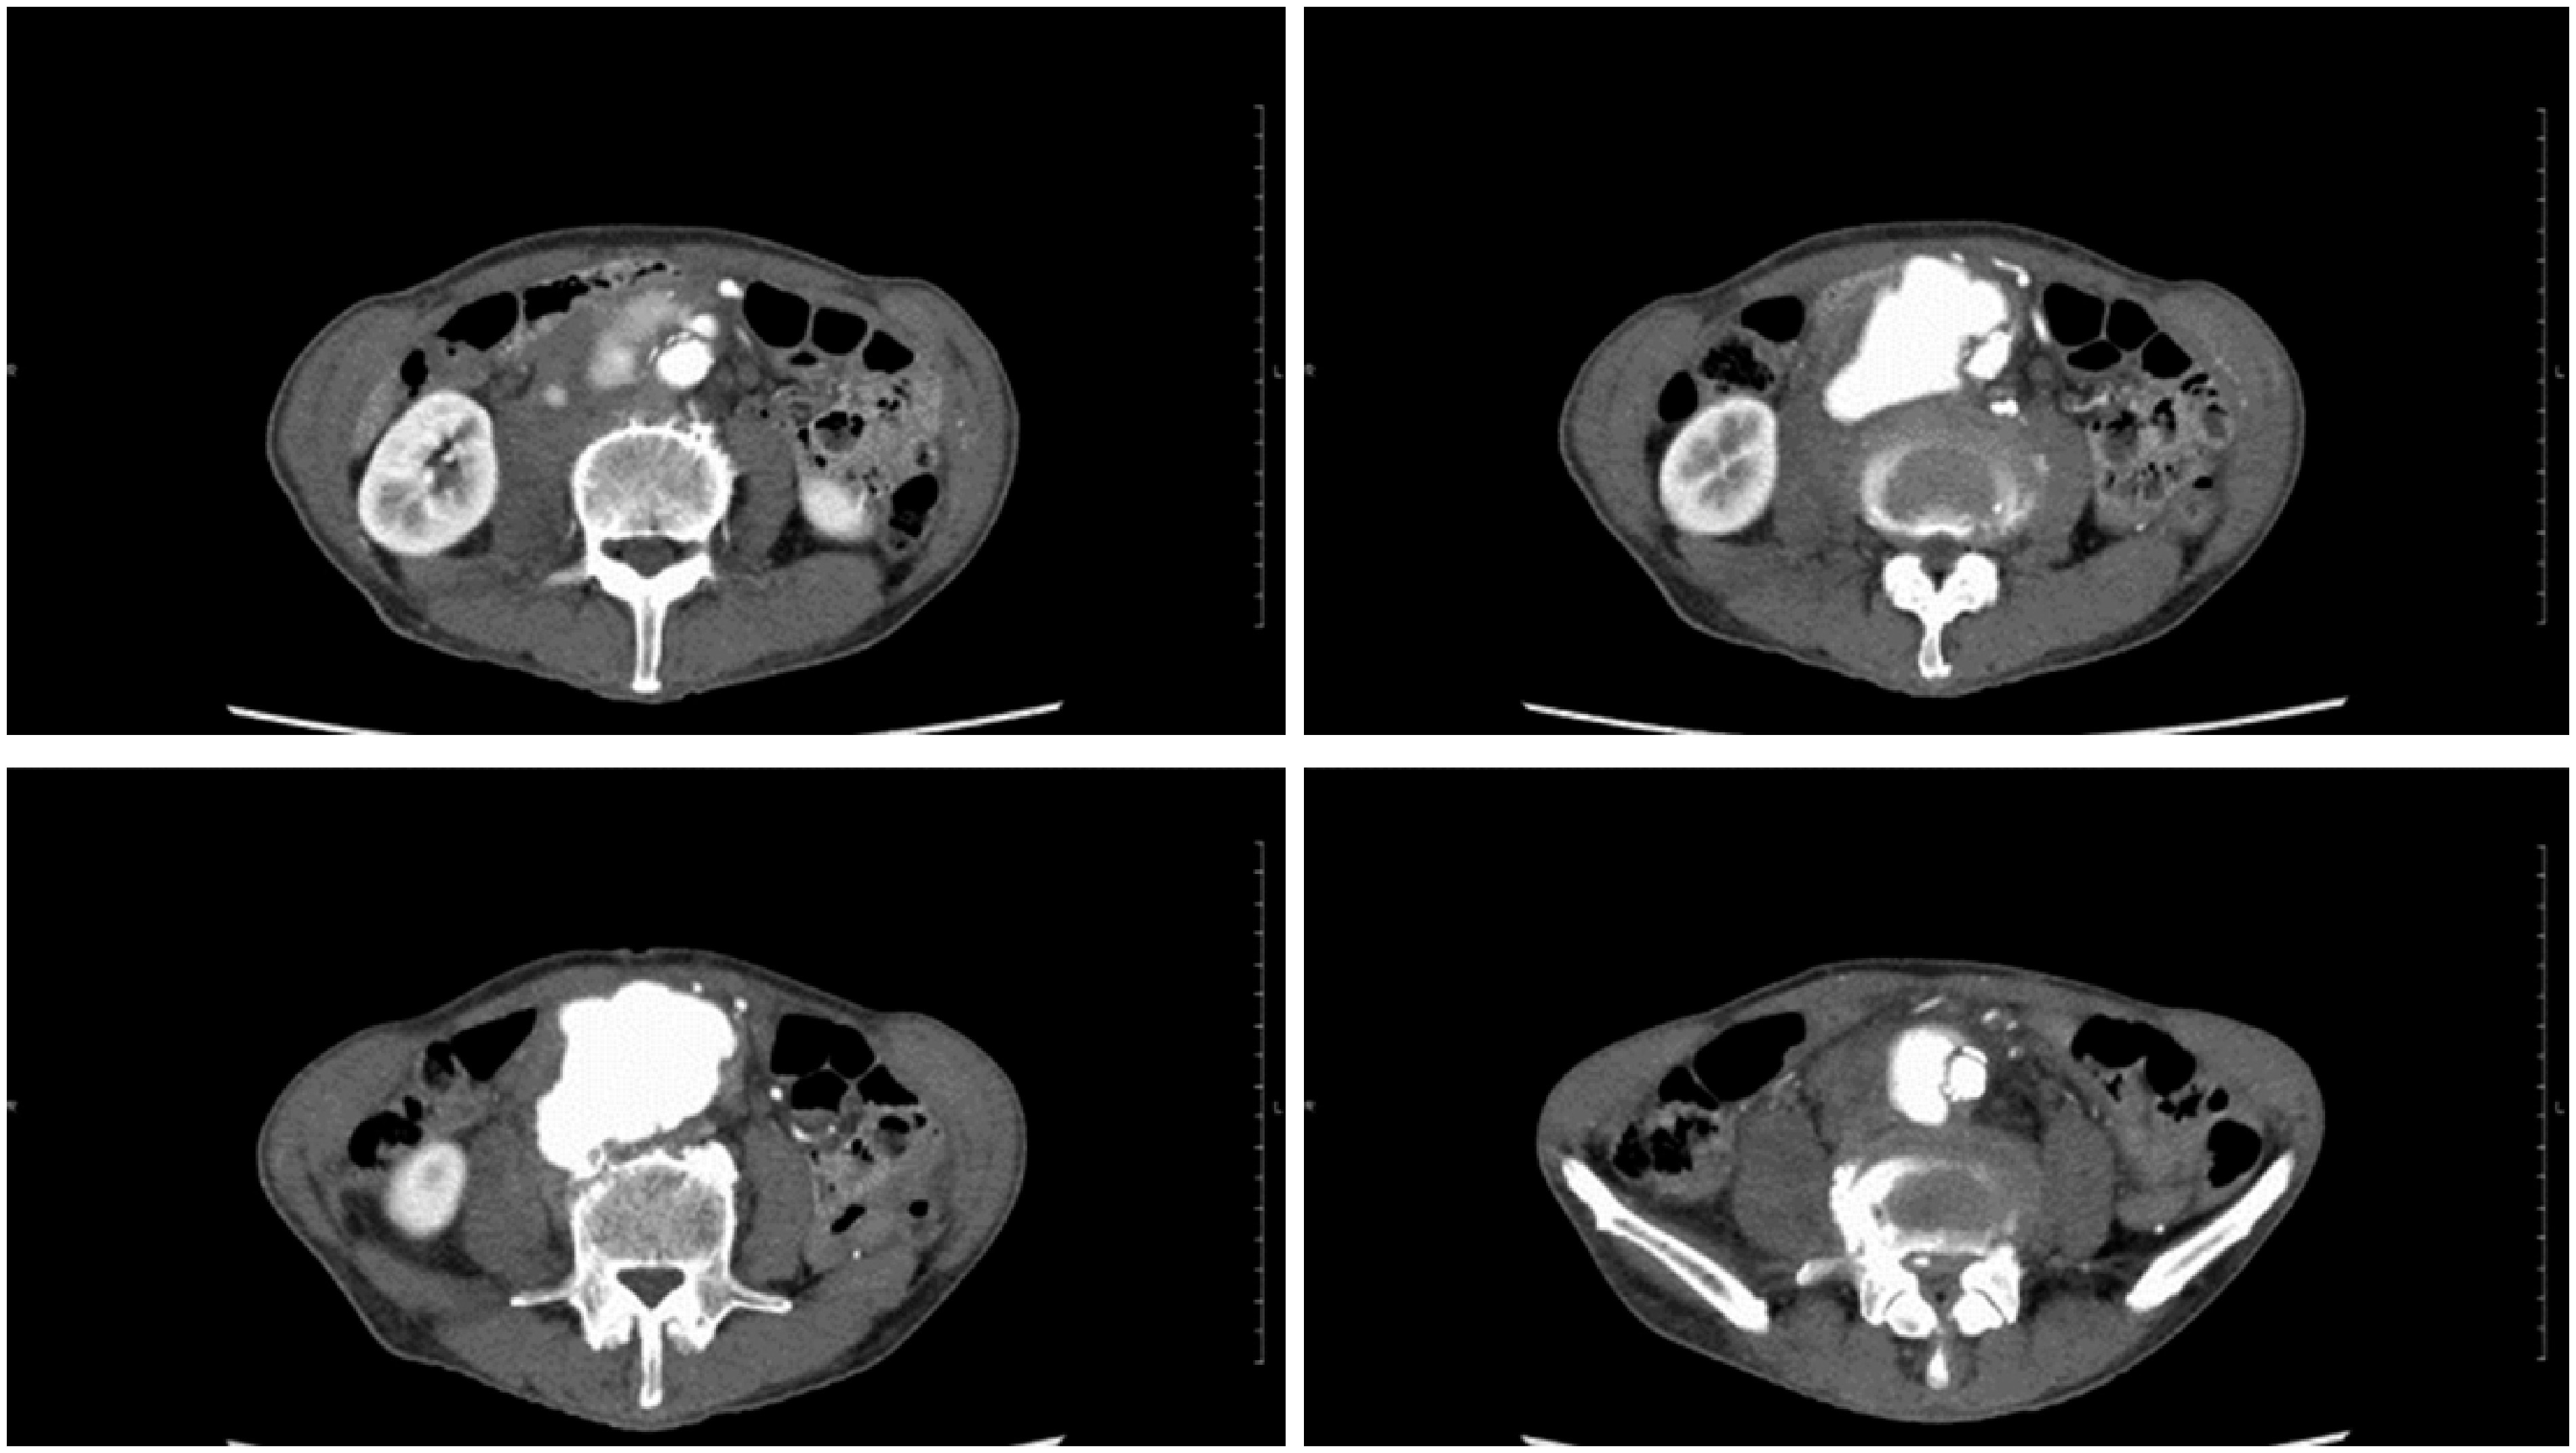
Fig. 1 A contrast-enhanced computed tomography scan revealed an abdominal aortic aneurysm with a saccular, multilocular appearance and an irregular border. The aneurysm extended from the infrarenal abdominal aorta to just above the bifurcation. Rupture of the aneurysm was suspected.
